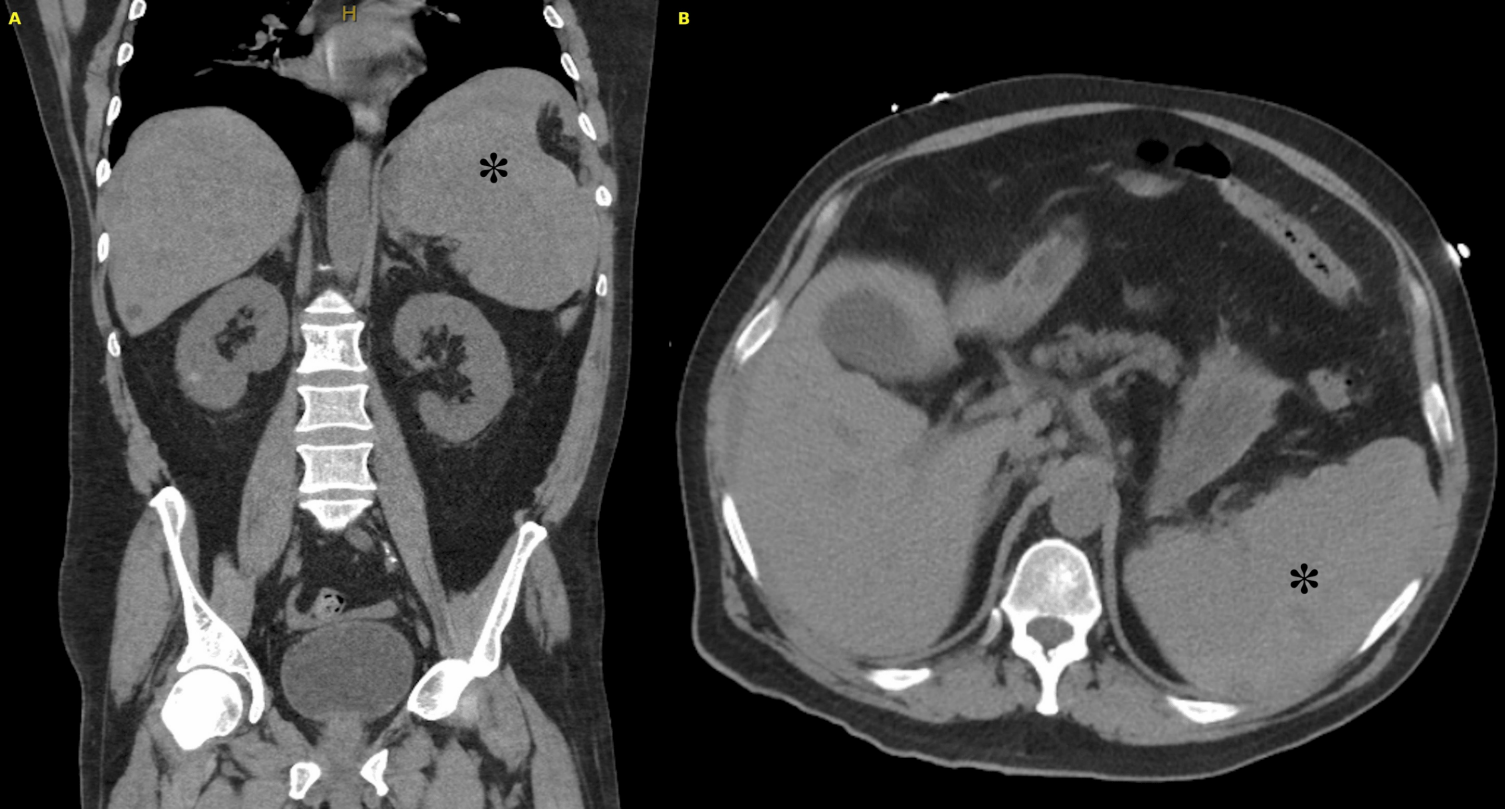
Preoperative CT images reveal markedly abnormal findings, with a large perisplenic hematoma obscuring differentiation between blood products and splenic parenchyma. Panel A (left) displays a coronal view of the spleen, while panel B (right) shows an axial view. An asterisk marks the ruptured spleen and the associated hematoma.
